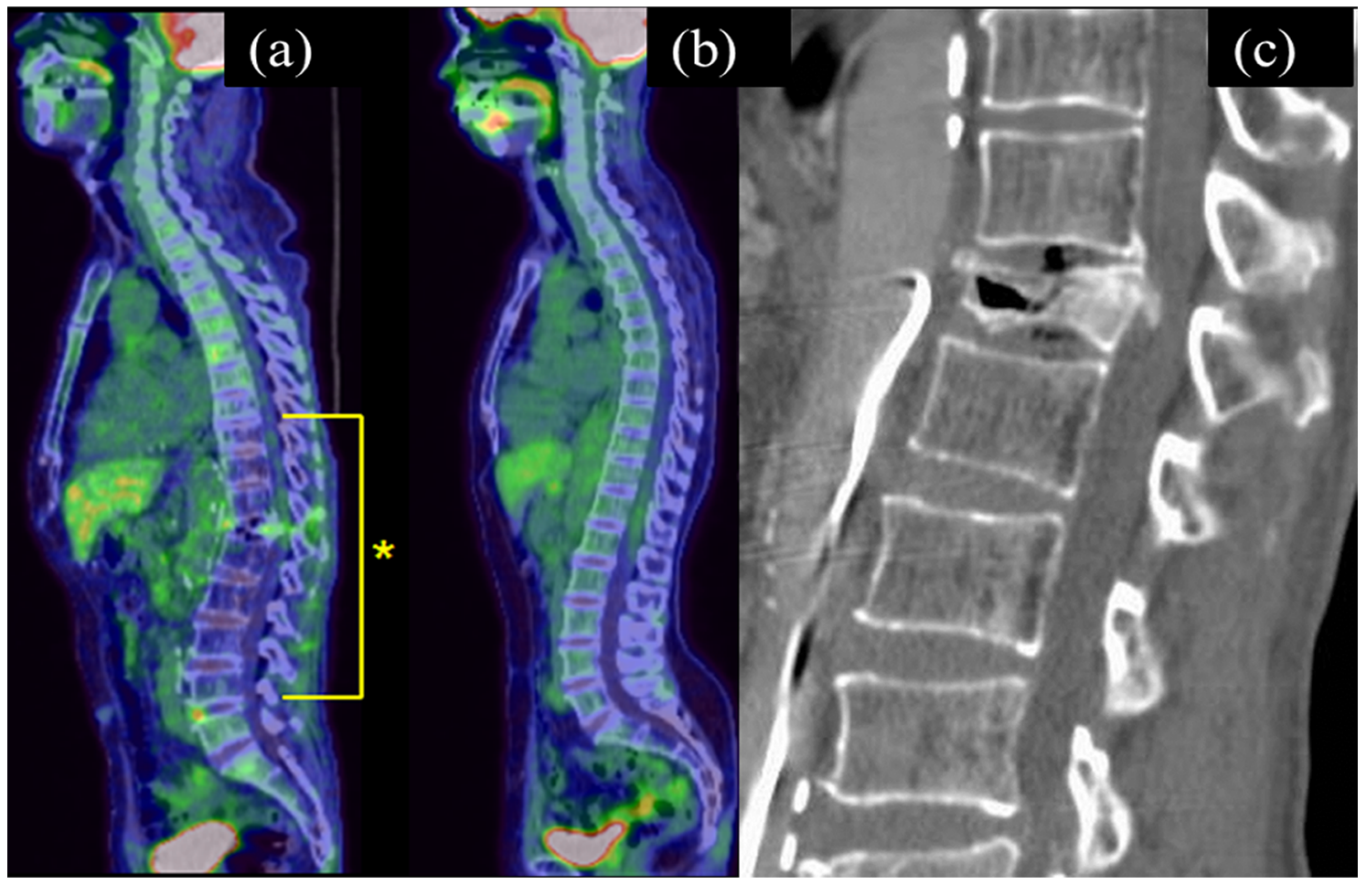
Sagittal reconstructed fluorodeoxyglucose-positron-emission tomography (FDG-PET)-CT image (a) and MDCT image (c) of the spine obtained from a 62-year-old man after eight transarterial chemoembolization procedures for hepatocellular carcinomas. FDG-PET-CT reveals diffusely decreased FDG uptake within the lower thoracic to lumbar spine (*). Sagittal reconstructed FDG-PET-CT image is accompanied by that of a 72-year old woman performed five years after resection of a uterine cervical cancer, in which there is normal spinal FDG uptake, for comparison (b). Sagittal reconstructed CT shows a vertebral fracture of the 12th thoracic vertebra.
